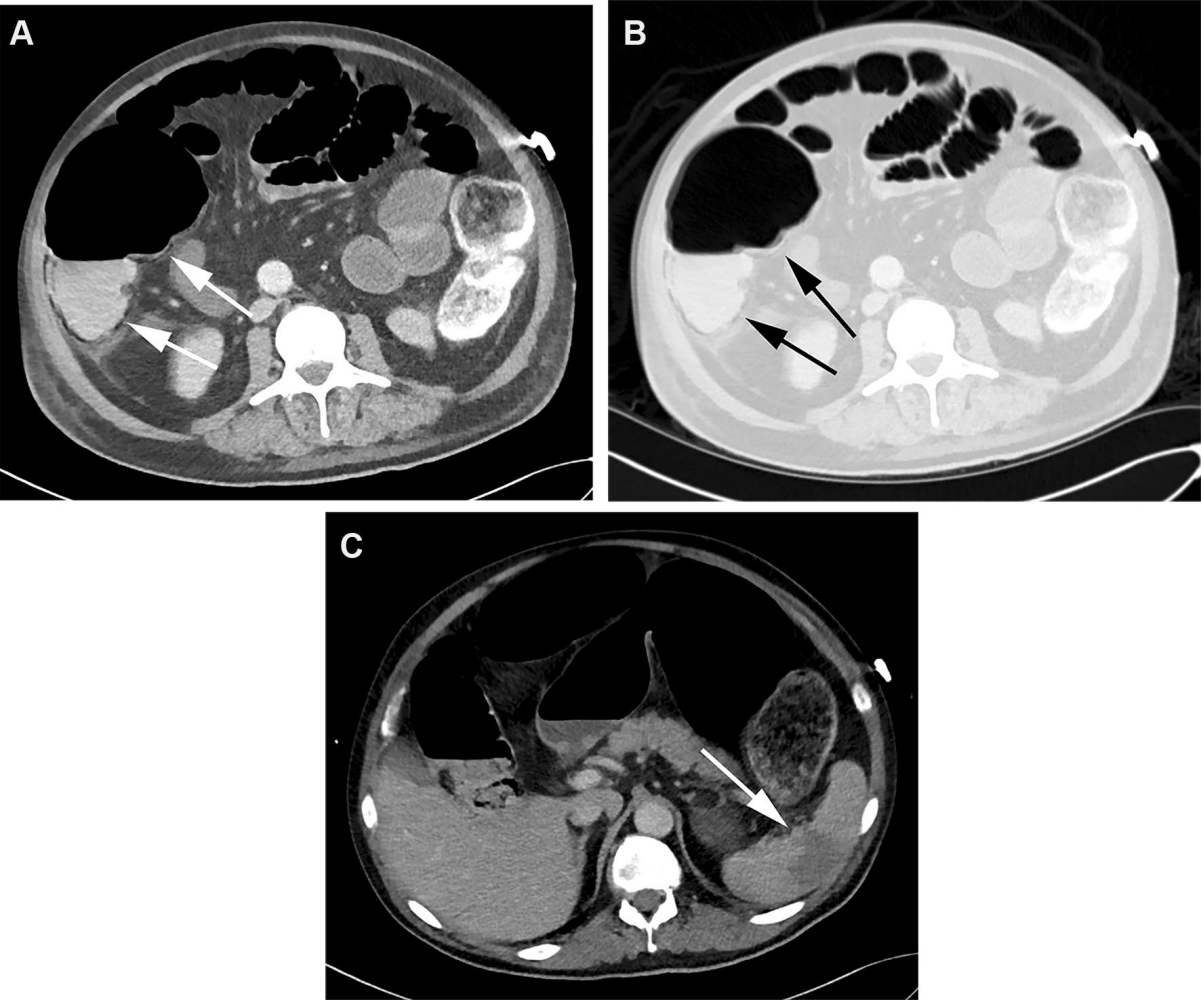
CT in the soft tissue (a) and lung window (b) of a 70-year-old male patient with PI in the colon (arrows) and distension of the small bowel and colon. In addition, perfusion inhomogeneities indicating ischemia in the spleen were found (c, arrow). The AST blood level was 80 IU/L (normal values 7–37 IU/L), the blood lactate level was 8.1 mmol/L (normal values 0.5–2.2 mmol/L). The patient died one day after CT
